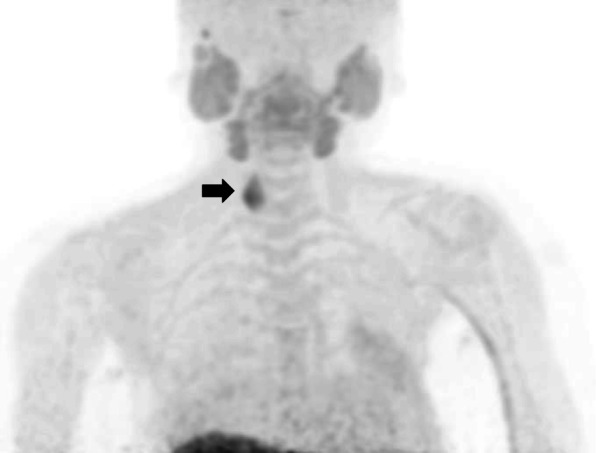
^11^C-methionine PET-CT findings after the removal of the upper and lower parathyroid glands. Methionine-positive tumor right next to the trachea (arrow)
